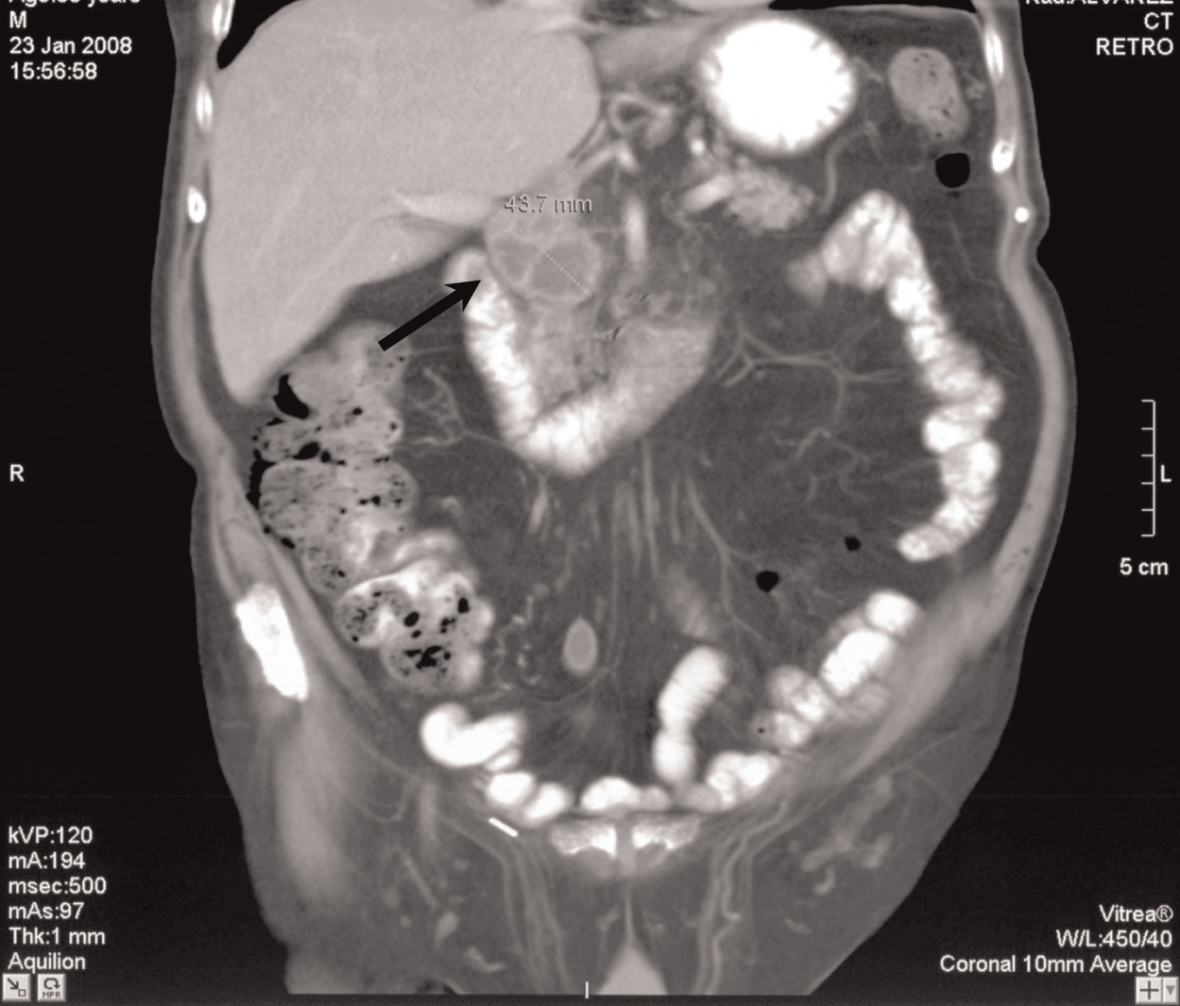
Computed tomography scan showing 43 mm abscess affecting the pancreatic head.
